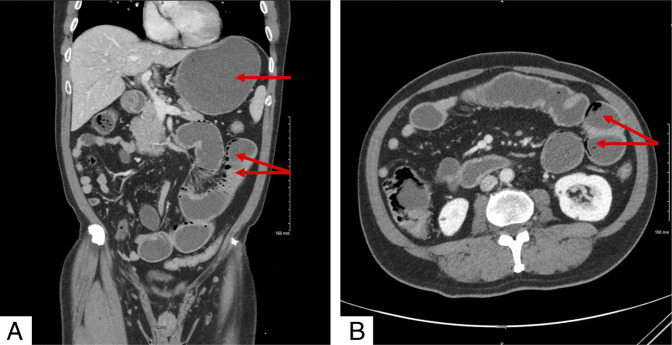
Computed tomographyof the abdomen and pelvis coronal (A) and axial (B) views showing pneumatosis intestinalis and marked distension of the stomach. The red arrows indicate the areas of interest.
